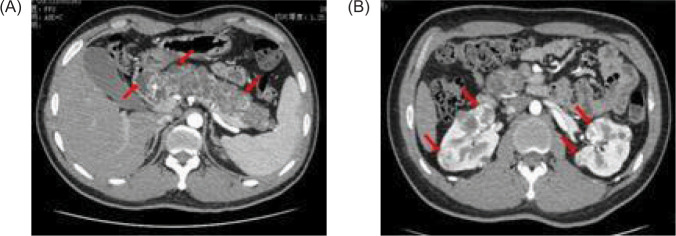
Contrast-enhanced CAT (CT) scan of the abdomen shows (A) multiple low-density nodules in the pancreas (red arrow); (B) low-density nodules in the left kidney, and multiple nodules of different sizes in both kidneys, which were heterogeneous enhancement (red arrow).
